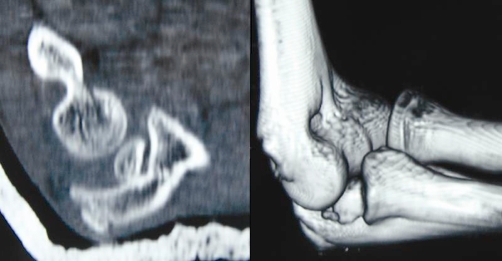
CT scan images. (A) Intraarticular fragment located into the humerocubital joint. (B) 3D reconstruction image. The fragment belonged to the medial epicondyle.
